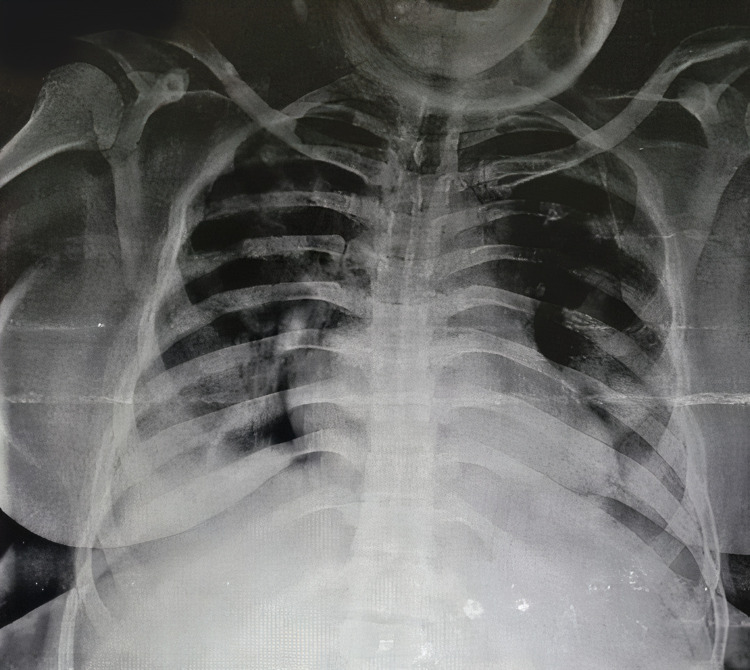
X-ray of case 1 after bevacizumab
